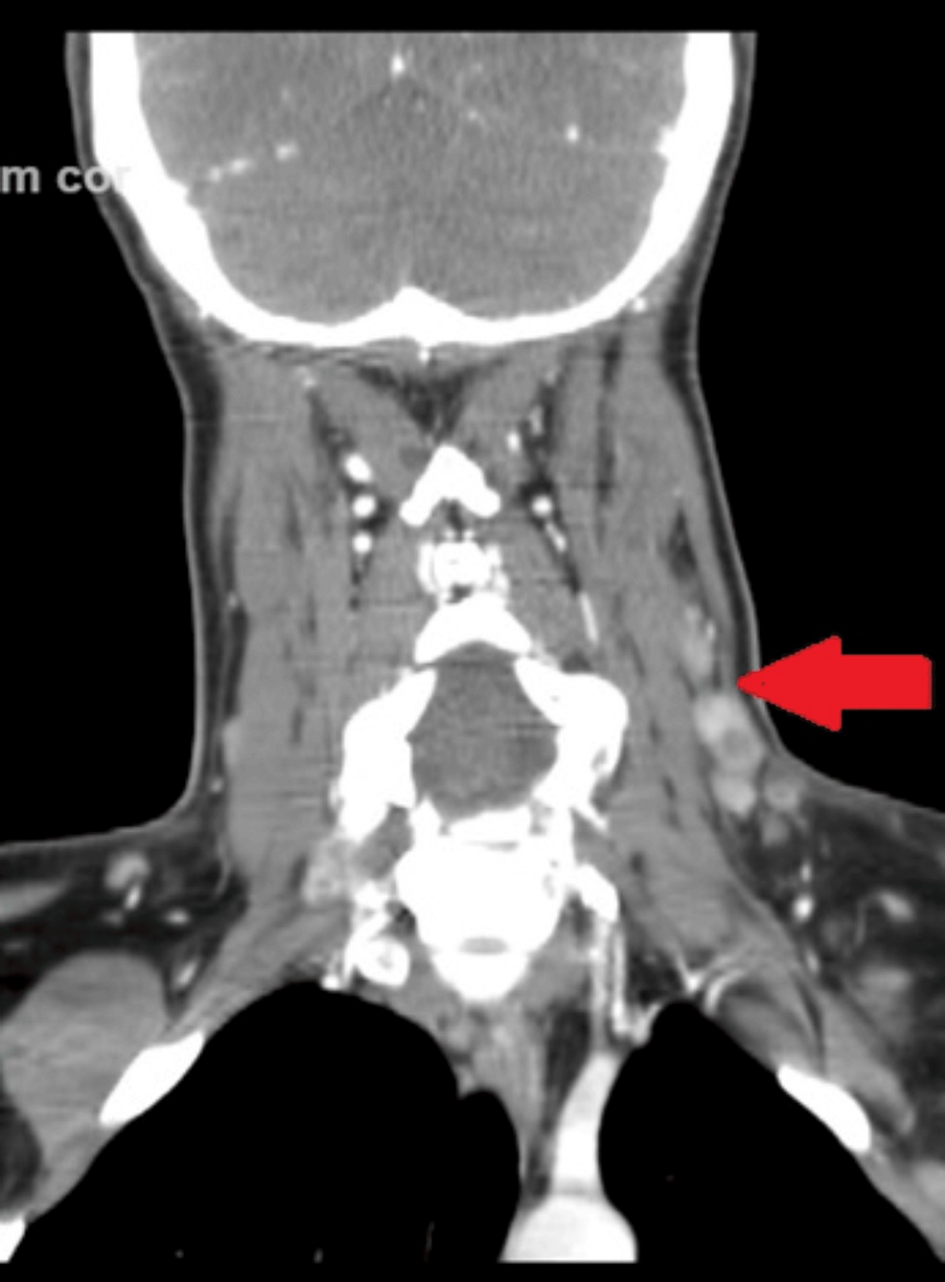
Computer tomography (CT) scan of the neck and soft tissues coronal section revealing multiple lymph node enlargements on the left posterior cervical chain (1.3x1 cm level 1 lymph node, 1x1 cm level 2 lymph node, 1.5x1 cm level 3 lymph node, red arrow)
